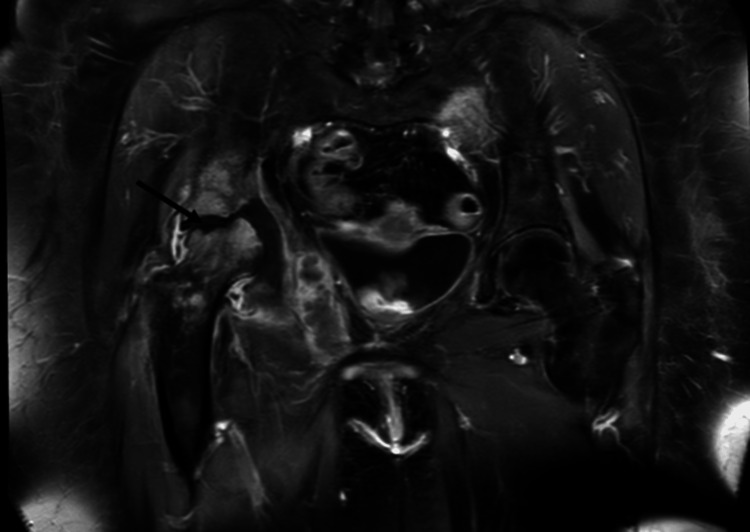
MRI of pelvis showing destroyed acetabular head (arrow)
